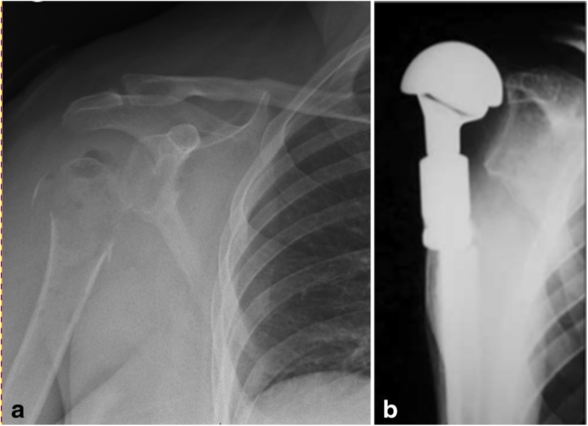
Radiographs of proximal humerus destruction by metastatic breast cancer (a) and after modular endoprosthetic replacement (b)
